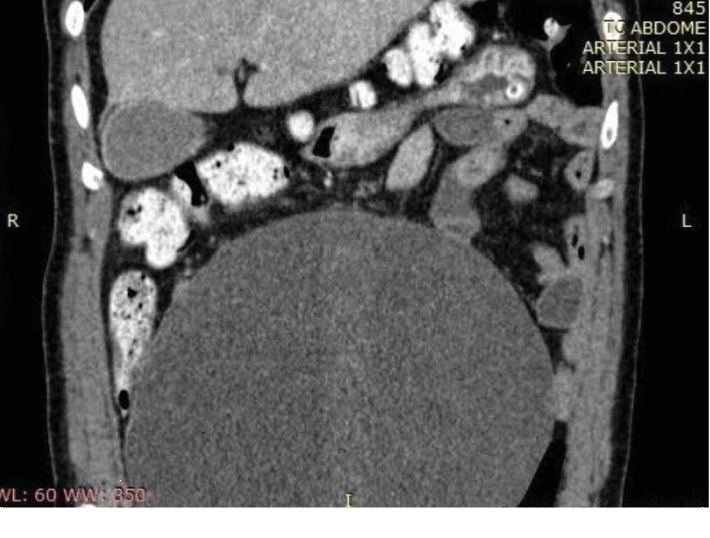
Computed tomography in coronal reconstruction.
